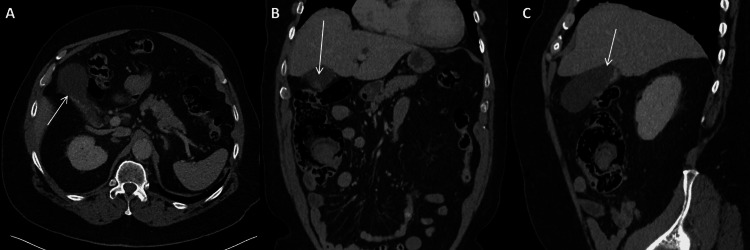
First Preoperative CT This is the first preoperative CT scan. The white arrows identify the gallbladder. Pane A shows the transverse section. Pane B shows the coronal section. Pane C shows the sagittal section.
